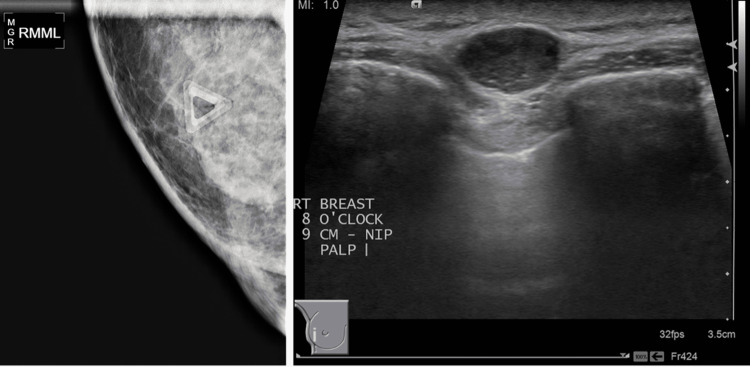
Mammogram and ultrasound findings (Left): Spot magnification medial-lateral mammogram of the right breast showing a 12 x 8 x 11 mm circumscribed lesion at the 8 o’clock location 9 cm from the nipple; (Right): Targeted right breast ultrasound showing the hypoechoic, well-circumscribed mass seen on the mammogram.
